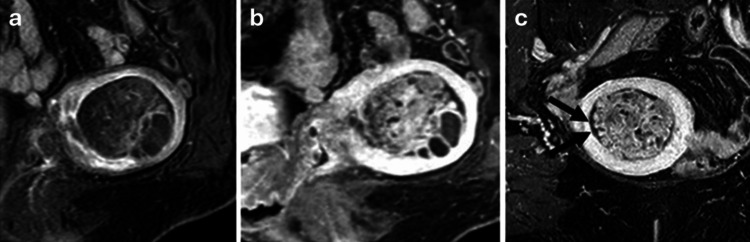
Contrast-enhanced MRI of the pelvis (a) In the early phase of the dynamic contrast-enhanced study (sagittal plane), the solid components of the mass exhibit heterogeneous enhancement. (b) In the delayed phase (sagittal plane), the mass shows prolonged enhancement. (c) The papillary surface of the mass is clearly visualized on thin-slice three-dimensional axially reformatted T1-weighted images after gadolinium administration (coronal plane, arrows). Panels (a) and (b) were selected to best demonstrate contrast enhancement patterns, whereas panel (c) was obtained from a different optimized slice and imaging plane to better depict papillary surface morphology; therefore, identical anatomical sections are not shown across panels MRI: magnetic resonance imaging
